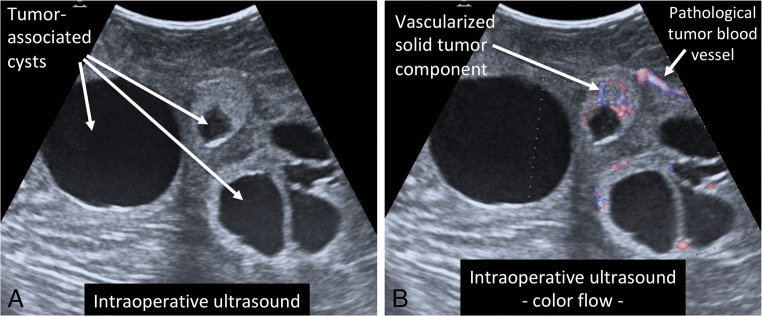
Intraoperative ultrasound. Intraoperative ultrasound showing multiple cerebellar hemangioblastomas with tumor-associated cysts (a). The color flow feature (b) allows visualization of the blood flow including the high vascularization of the solid tumor component as well as pathological blood vessels supplying the hemangioblastoma
